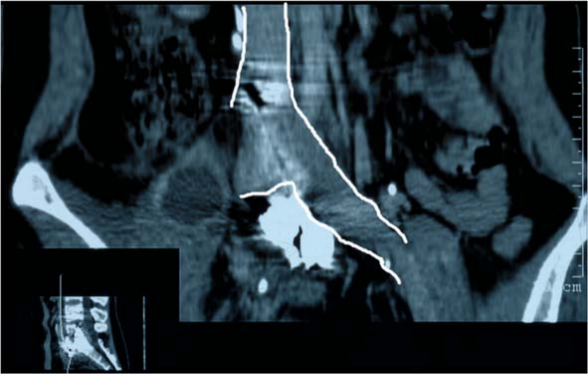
Postoperative computed tomographic angiography (CTA) showing iliac vein adjacent to the lumbosacral spine (outlined by white lines) at the 24 months’ follow up
